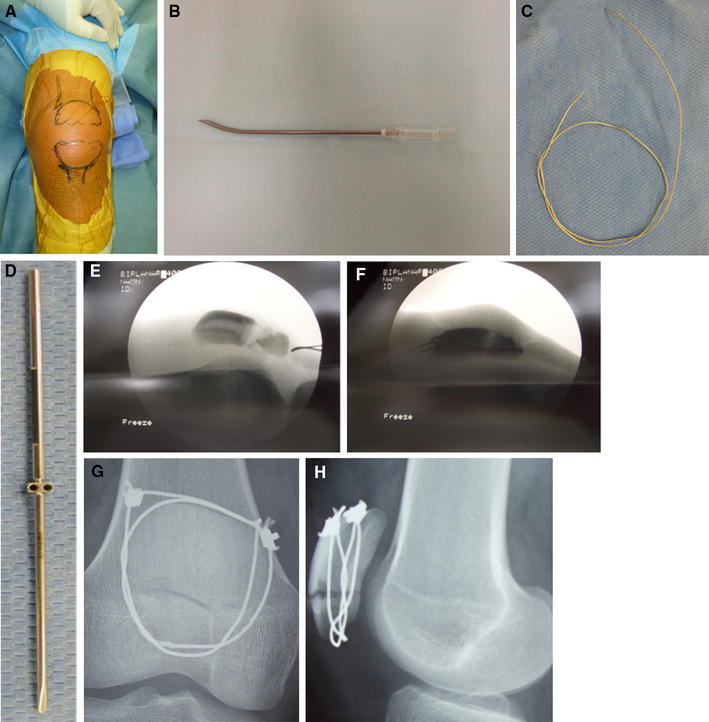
Surgical technique. a Ten-millimetre and 5-mm small medio-lateral skin incisions at the superior and inferior margins of the patella, respectively. b The tip of the inner tube of a Sur-flo (HAKKO ELASTER TYPE-1, HAKKO Co., Ltd., Tokyo, Japan) was bent and used as a cable passer. c Cable, d sleeve: for these, the AI-wiring system (AI-medic Co., Ltd., Tokyo, Japan) was used. e The cable passer was inserted into the patellar tendon through the skin incision at the inferior margin of the patella. f The cable was applied around the patella including the soft tissues, penetrating through the quadriceps femoris tendon on the proximal side, and then passed along the superior margin of the patella. g Frontal view after fixation on plain X-ray radiography, h lateral view after fixation on plain X-ray radiography: two cable wires were passed through the shallow and deep layers, respectively
